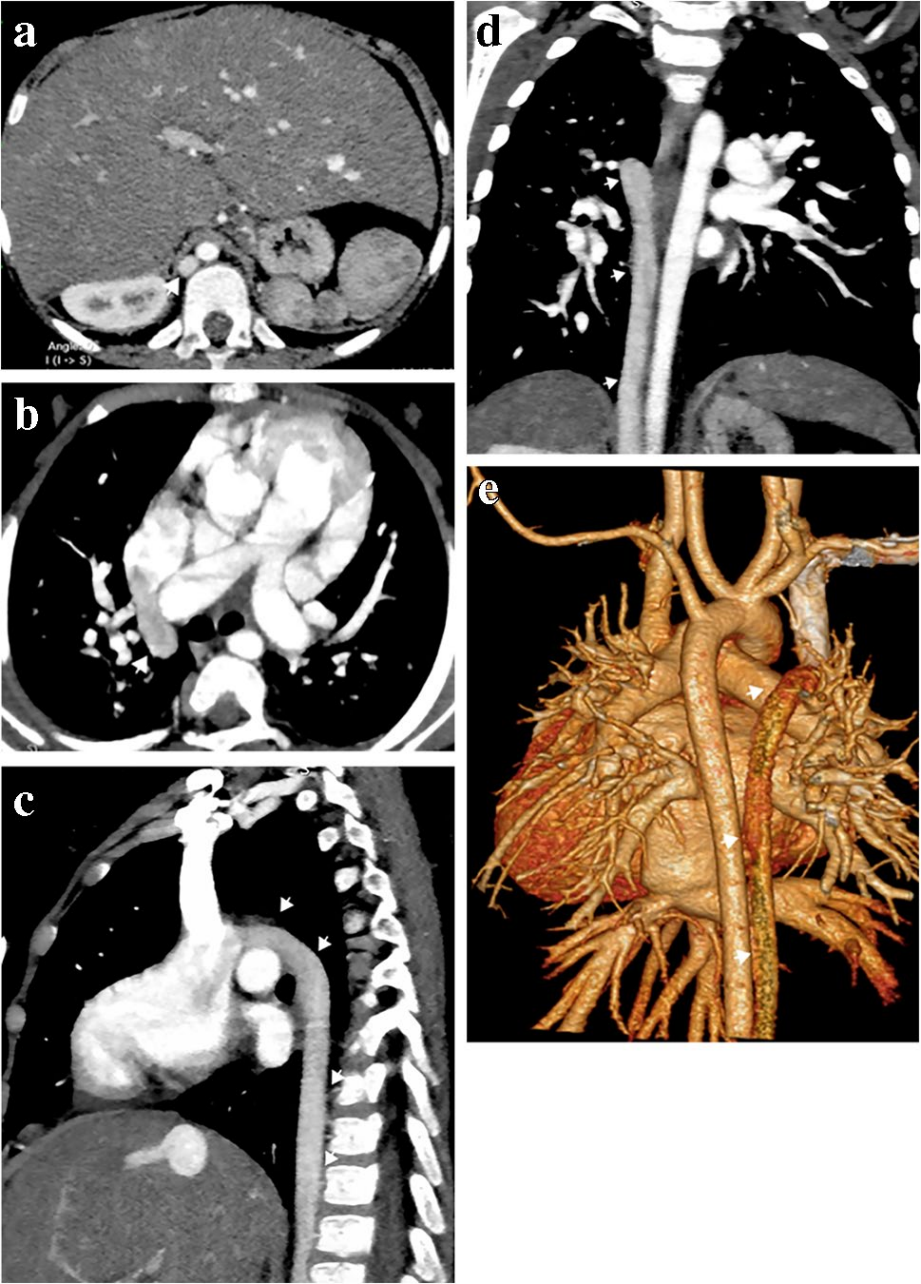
Inferior vena cava (IVC) interruption with azygos continuation in a 9-month-old boy with left isomerism. a–e Axial (a, b), sagittal (c) and coronal oblique (d) computed tomography (CT) images and volume-rendered CT image (e) show IVC interruption with azygos continuation (*arrows*) draining through the azygos arch into the superior vena cava (SVC). Note the polysplenia on the left side and central liver position
